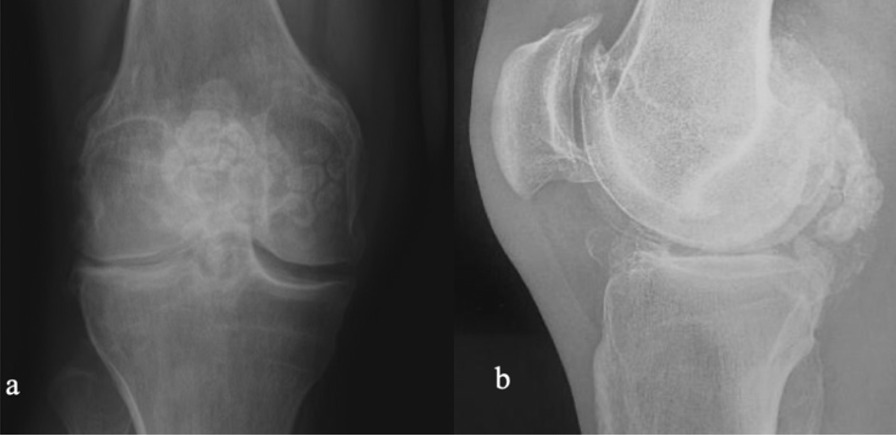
a Anterior–posterior and b lateral radiographs of the right knee. Multiple loose bodies in the posterior compartment
